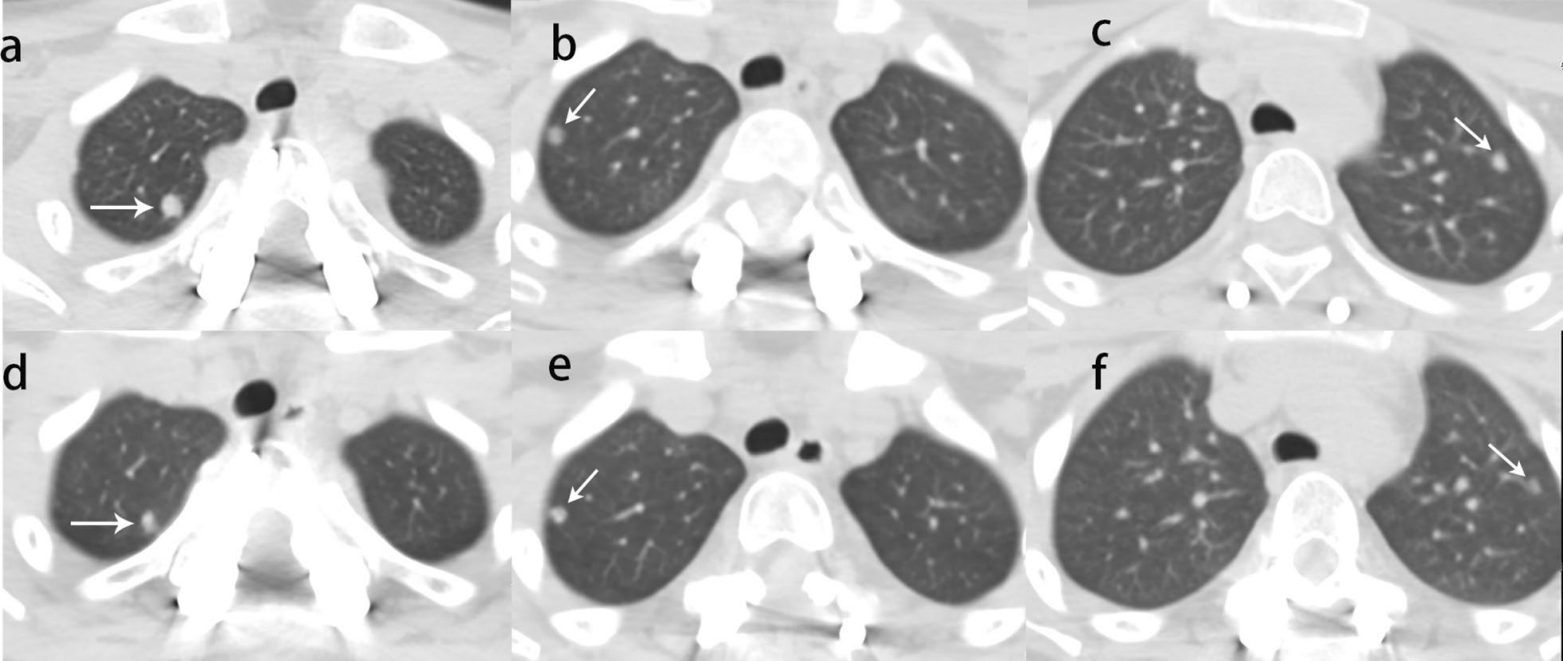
Image of giant cell tumor of T2 in a 13-year-old woman. a, b, c Pre-treatment CT scans showed three lung metastases and the largest diameters was 6.17 mm, 7.19 mm, and 7.92 mm (white arrow). d, e, f After denosumab treatment, lung metastases shrank to 5.09 mm, 6.32 mm, and 5.58 mm, respectively (white arrow)
